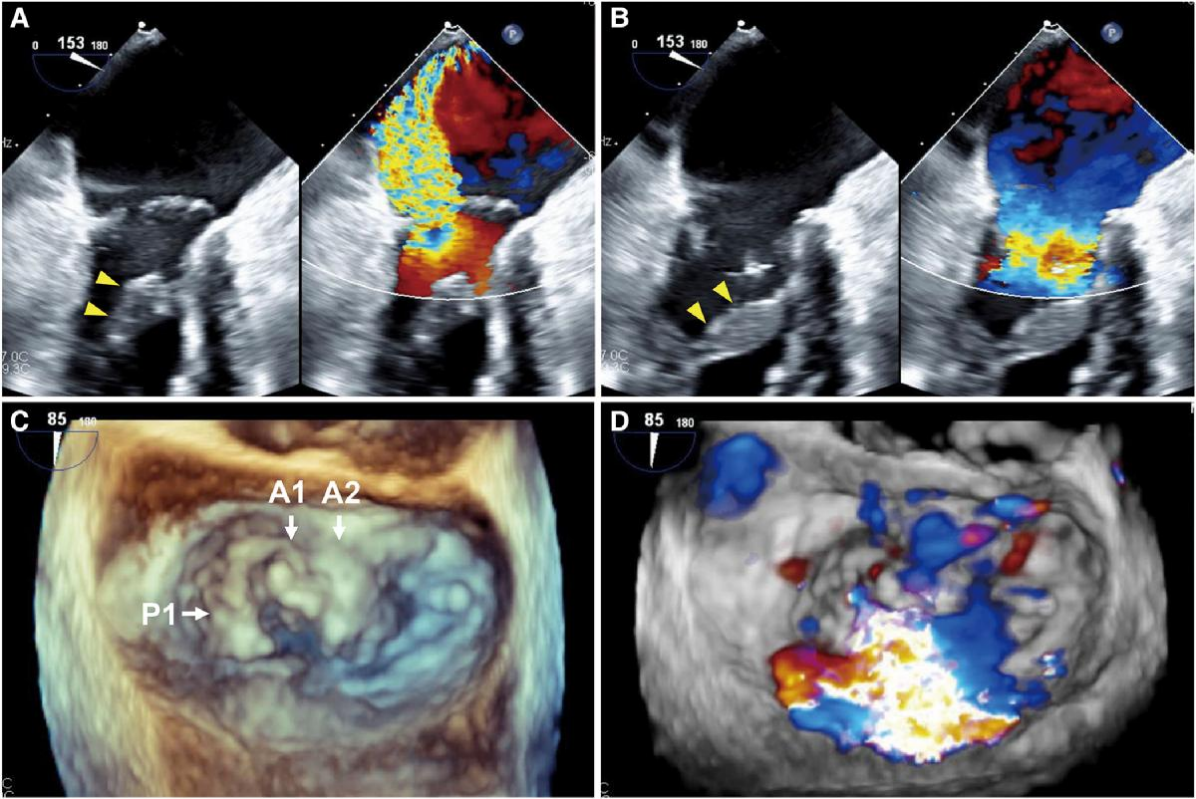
Preoperative echocardiography. (*A*) Severe mitral regurgitation due to A1/A2 segment prolapse with hypertrophied anterior papillary muscle (arrowheads) at systole. (*B*) Hypertrophied anterior papillary muscle (arrowheads) at diastole. (*C*) 3D image of the mitral valve. (*D*) 3D colour image of the mitral valve.
